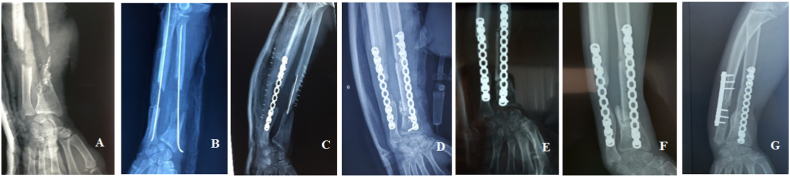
A. X-ray on admission: fractures of the middle 1/3-lower 1/3 junction of the ulna and radius with PSO/B. Control X-ray after Metaizeau pinning/C. Rx after cancellous grafting of the ulna and placement of the cement for the radius/D. Postoperative T2 Masquelet Rx/E. Rx at 5 months/F. Rx at 7 months/G. Rx after shortening osteotomy.
